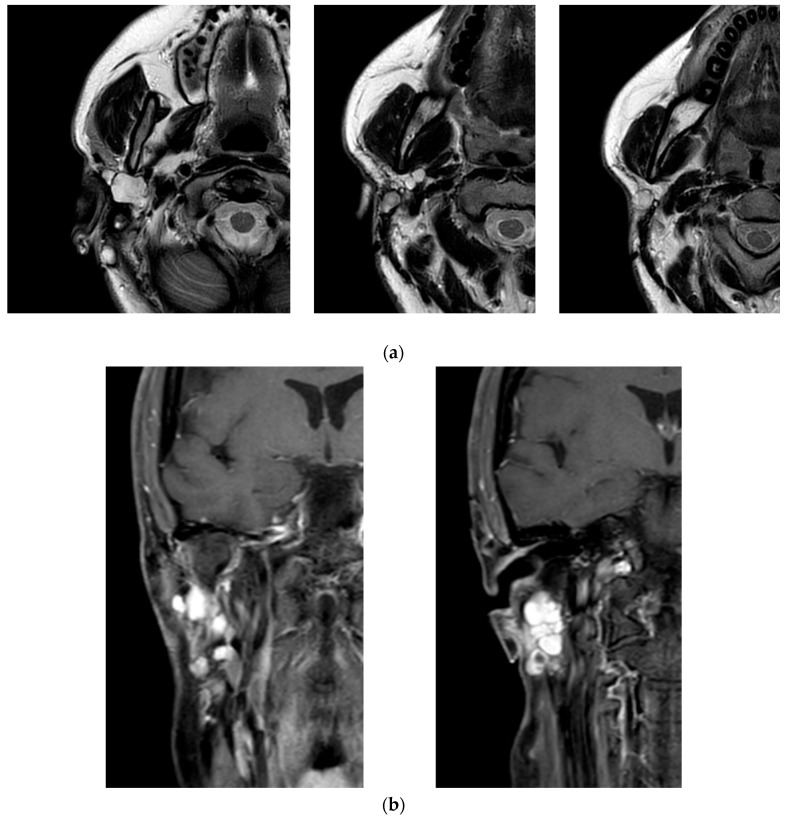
Magnetic resonance imaging (MRI) of the head and neck performed during the most recent recurrence of the pleomorphic adenoma. (a) Axial T2-weighted image showing a well-circumscribed, multilobulated lesion in the deep portion of the right parotid, with hyperintense signal and clear demarcation from adjacent structures. (b) Coronal contrast-enhanced T1-weighted image revealing intense peripheral enhancement of the lesion, consistent with a recurrent multinodular pleomorphic adenoma.
